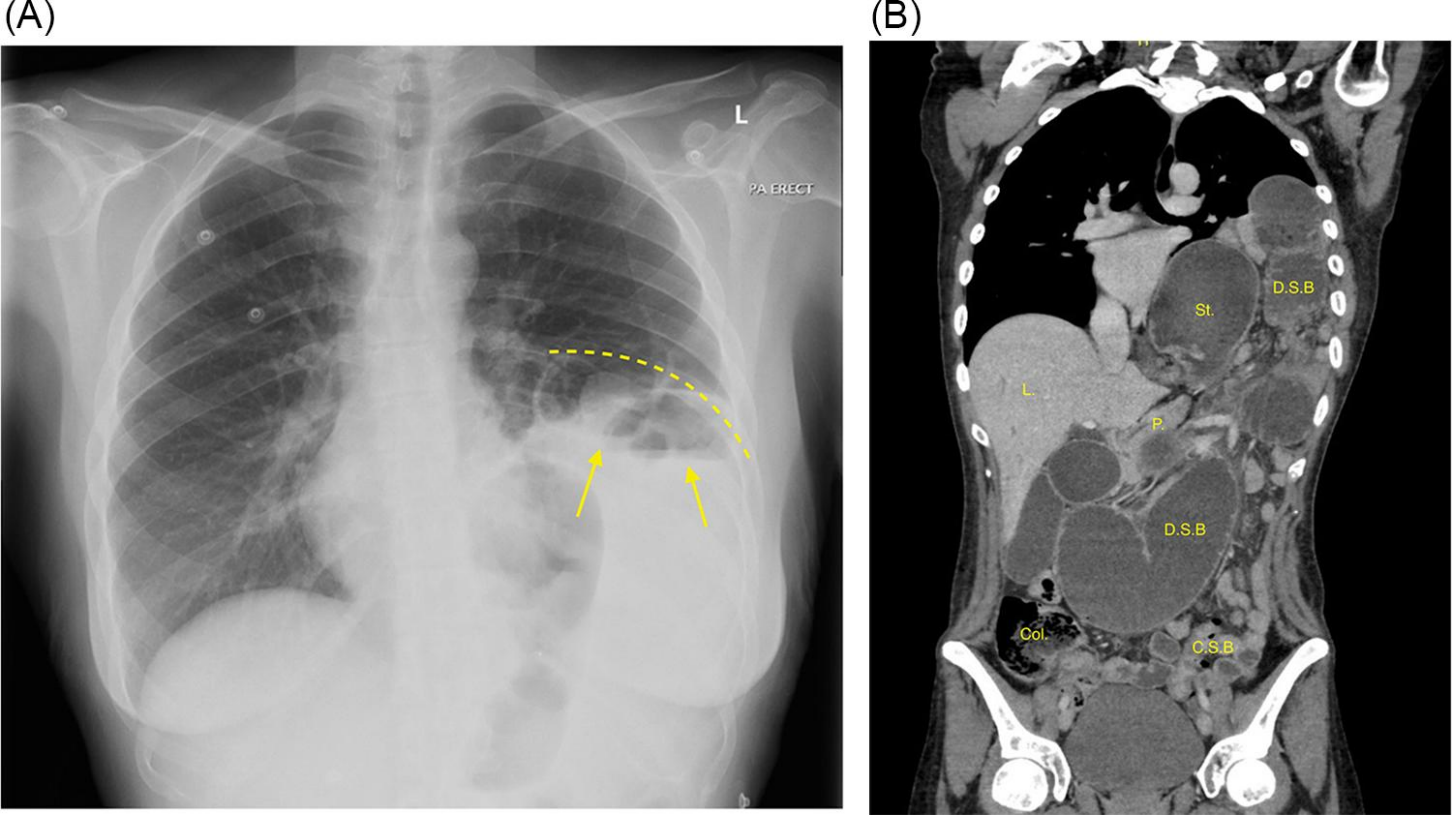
(A) Chest x-ray, PA view. Showing large left diaphragmatic hernia with obstruction, demonstrated by herniated small bowel loops with air-fluid levels (arrows) (B) CT scan of the chest, abdomen, and pelvis with contrast, coronal view. Showing large left diaphragmatic hernia containing a dilated stomach, dilated small bowel loop and collapsed small bowel loops. (L.;liver, P.;pancreas, St.;stomach, Col.;colon, D.S.B; dilated small bowels, C.S.B.; collapsed small bowels).
